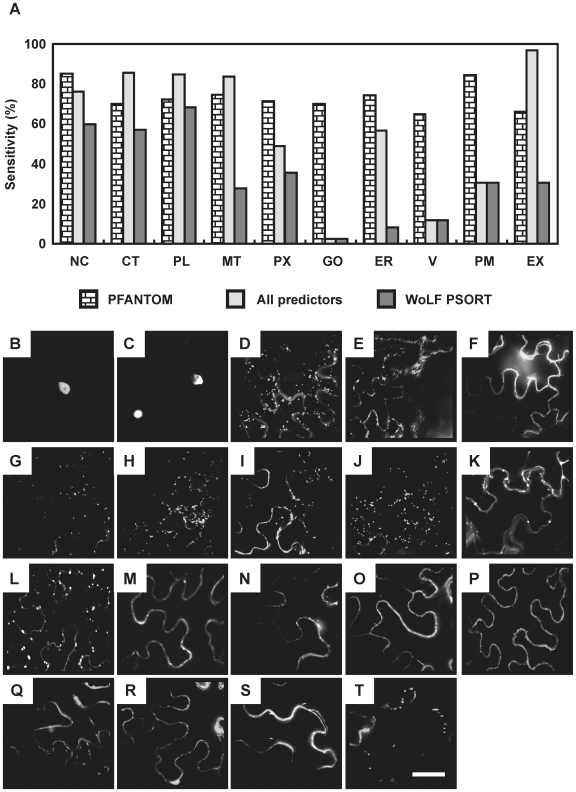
Validation of the PFANTOM method for plant subcellular localizations. (A) Comparison of the prediction performance of Pfam-based prediction (PFANTOM), all predictors by SUBA database, and WoLF PSORT. NC, nucleus; MT, mitochondrion; V, vacuole; PX, peroxisome; ER, endoplasmic reticulum; GO, Golgi apparatus; CT, cytosol; PM, plasma membrane; PL, plastid; EX, extracellular. (B-T) Subcellular localization of transiently expressed YFP-fusion proteins in *N. benthamiana*. (B) bHLH (AT5G48560); (C) SND1 (AT1G32770); (D) Golgi marker (STtmd-GFP); (E) ER marker (GFP-HDEL); (F) Plasma membrane marker (pm-rk); (G) PGSIP1 (AT3G18660); (H) PGSIP3 (AT4G33330); (I) TBL3 (AT5G01360, belonging to DUF231); (J) Unknown protein (AT2G38320, belonging to DUF231); (K) CTL2 (AT3G16920); (L) FLA11 (AT5G03170); (M) FLA12 (AT5G60490); (N) COBL4 (AT5G15630, IRX6); (O) LRR protein (AT1G67510); (P) NCRK (AT2G28250); (Q) RIC2 (AT1G27380); (R) RIC4 (AT5G16490); (S) ROP7 (AT5G45970); (T) ROPGEF4 (AT2G45890). A summary of the localization experiments is shown in Table 2. Scale bar = 20 µm.
